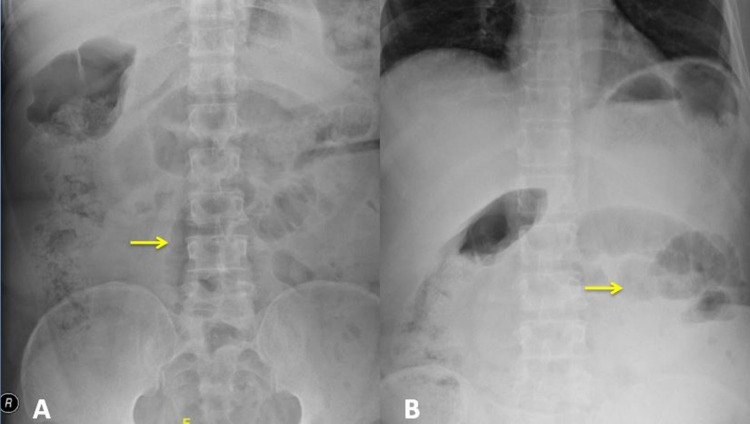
Abdominal X-ray showing (A) multiple dilated air small bowel loops (arrow) and (B) multiple air-fluid levels (arrow)
